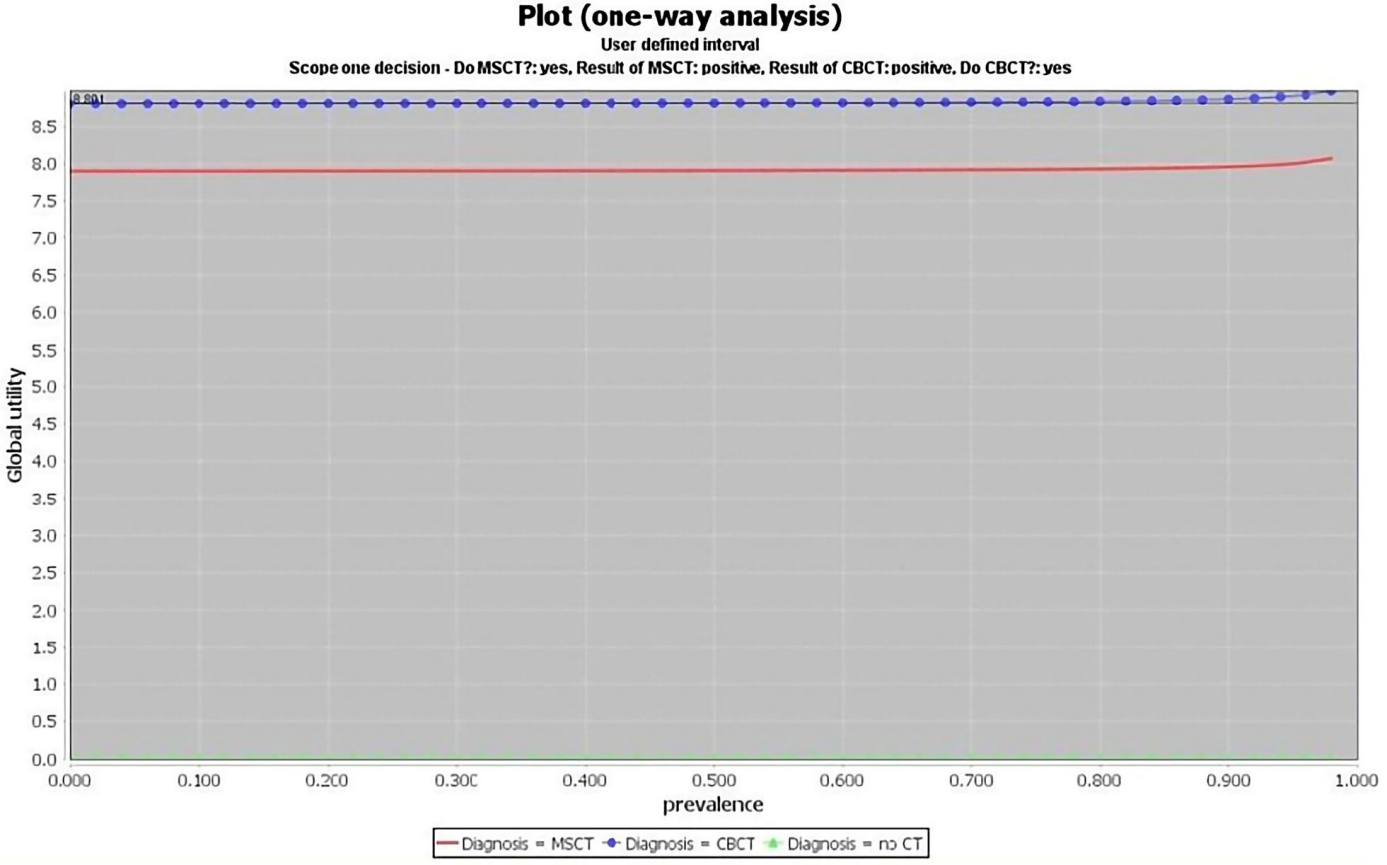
One-way analysis plot for the decision “Diagnosis”. The black horizontal line denotes the expected utility in the reference case. The red line denotes the effectiveness of MSCT, the blue line the effectiveness of CBCT, and the green line the effectiveness without CT. Effectiveness results 8.18 for MSCT and 9.08 for CBCT; the latter is the best choice
